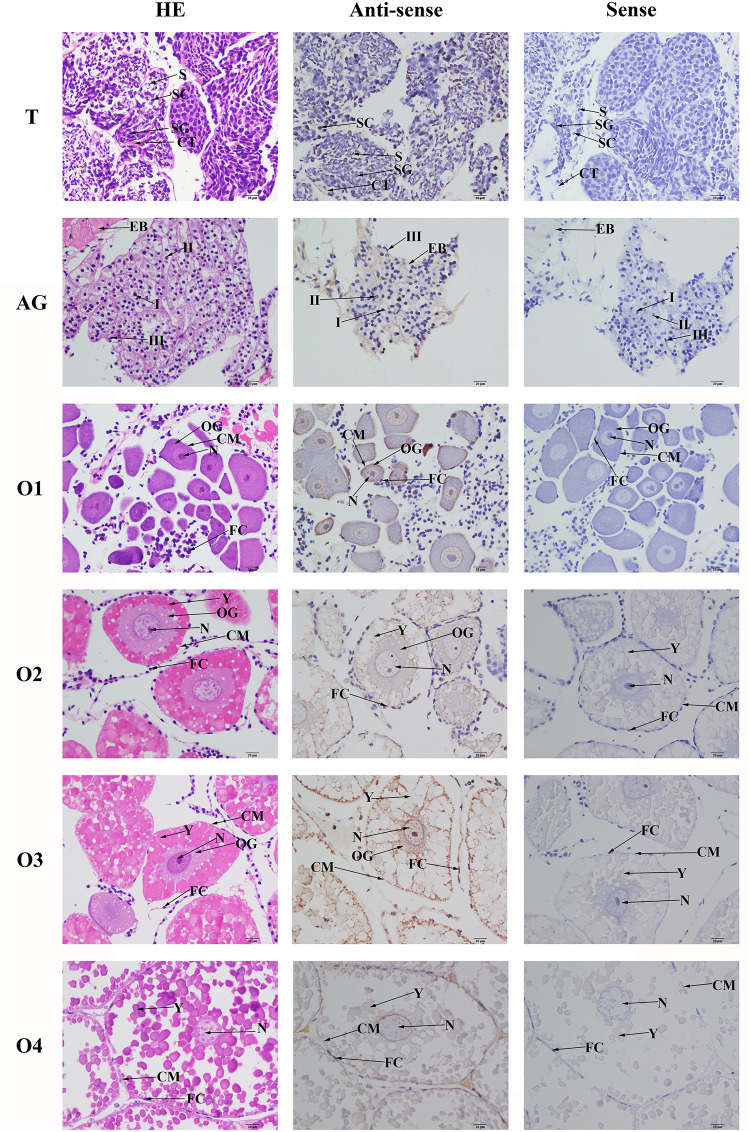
In situ* hybridization analysis of *Mn-SDHB* gene in testis and androgenic gland from reproductive season, and different ovarian reproductive cycle of *M*. *nipponense*. ST, seminiferous tubule; SG, Spermatogonia; SC, spermatocyte; S, sperm; CT, collecting tissue; E, wall epithelium; EM, eosinophilic matrix; VD, vas deferens; EB, ejaculatory bulb; OG, oogonium; OC, oocyte; CM, cytoplasmic membrane; N, nucleus; Y, yolk granule; FC, follicle membrane. Scale bars = 20 μm.
